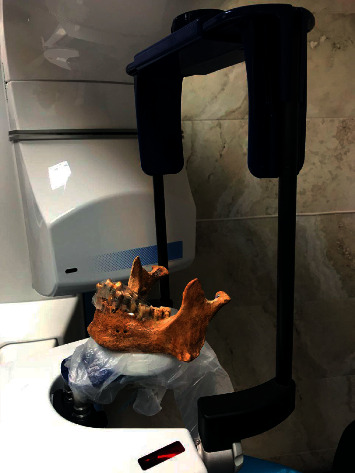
Teeth mounted on a dried mandible bone in the CBCT unit.
